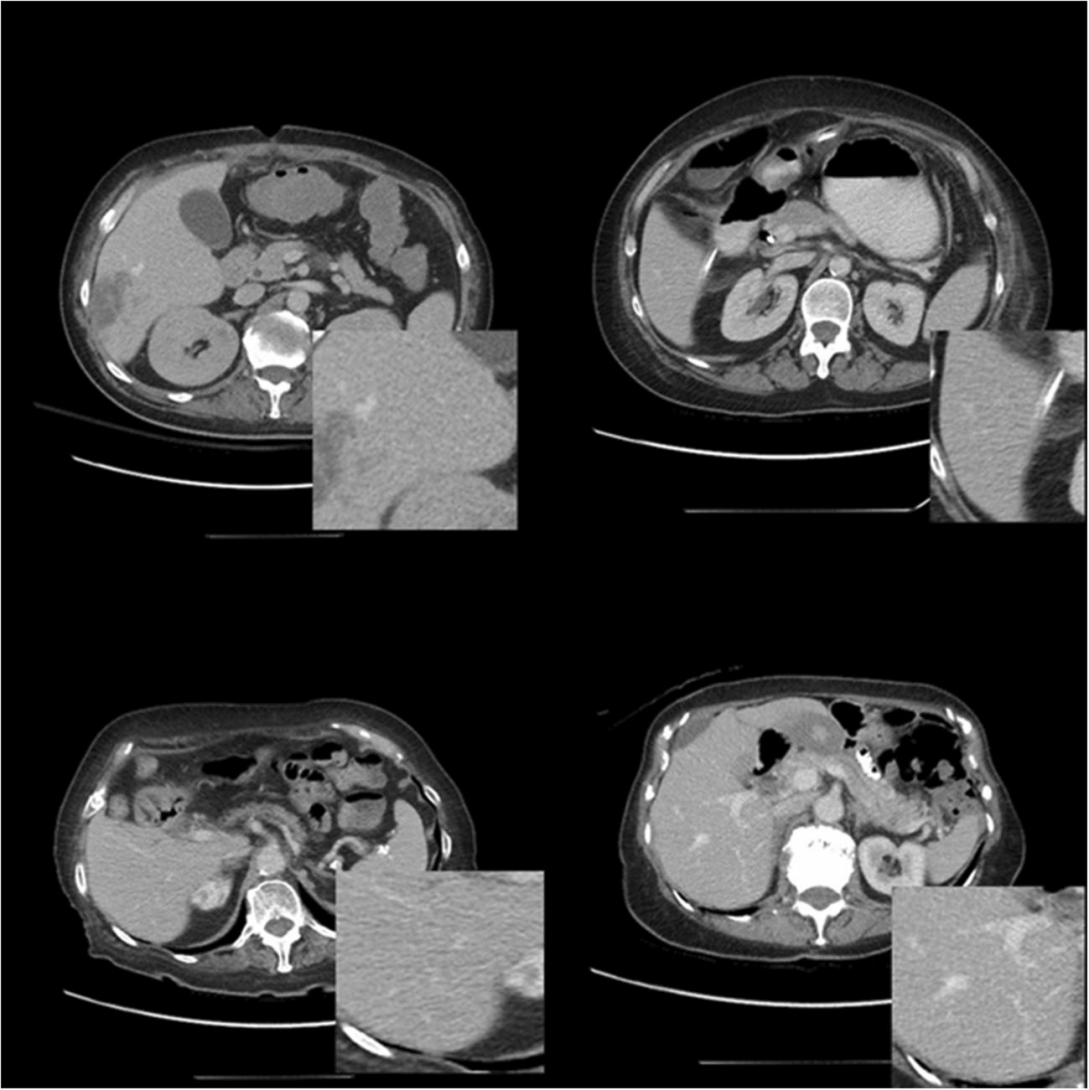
Abdominal CT images of different real-life patients, each effective diameter 310 mm. The figure depicts images with varying levels of kV and mAs and demonstrates that manipulation of either kV alone, mAs alone, or kV and mAs together are all possible means of optimizing radiation dose versus image quality. Acquisition parameters are as follows: top left – 120 kV, 206 mA; bottom left – 120 kV, 103 mAs; top right – 120 kV, 121 mAs; bottom right – 100 kV, 124 mAs
